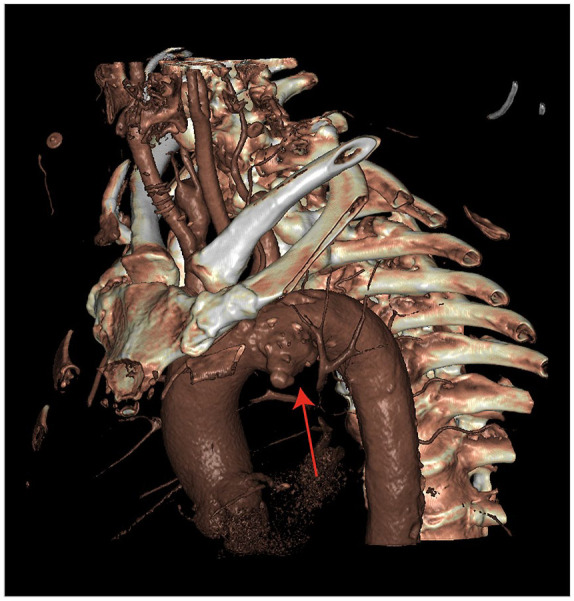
Three-dimensional (3D) rendering of part of the preoperative computed tomography (CT) volume, showing the saccular aneurysm in the aortic arch (red arrow).
